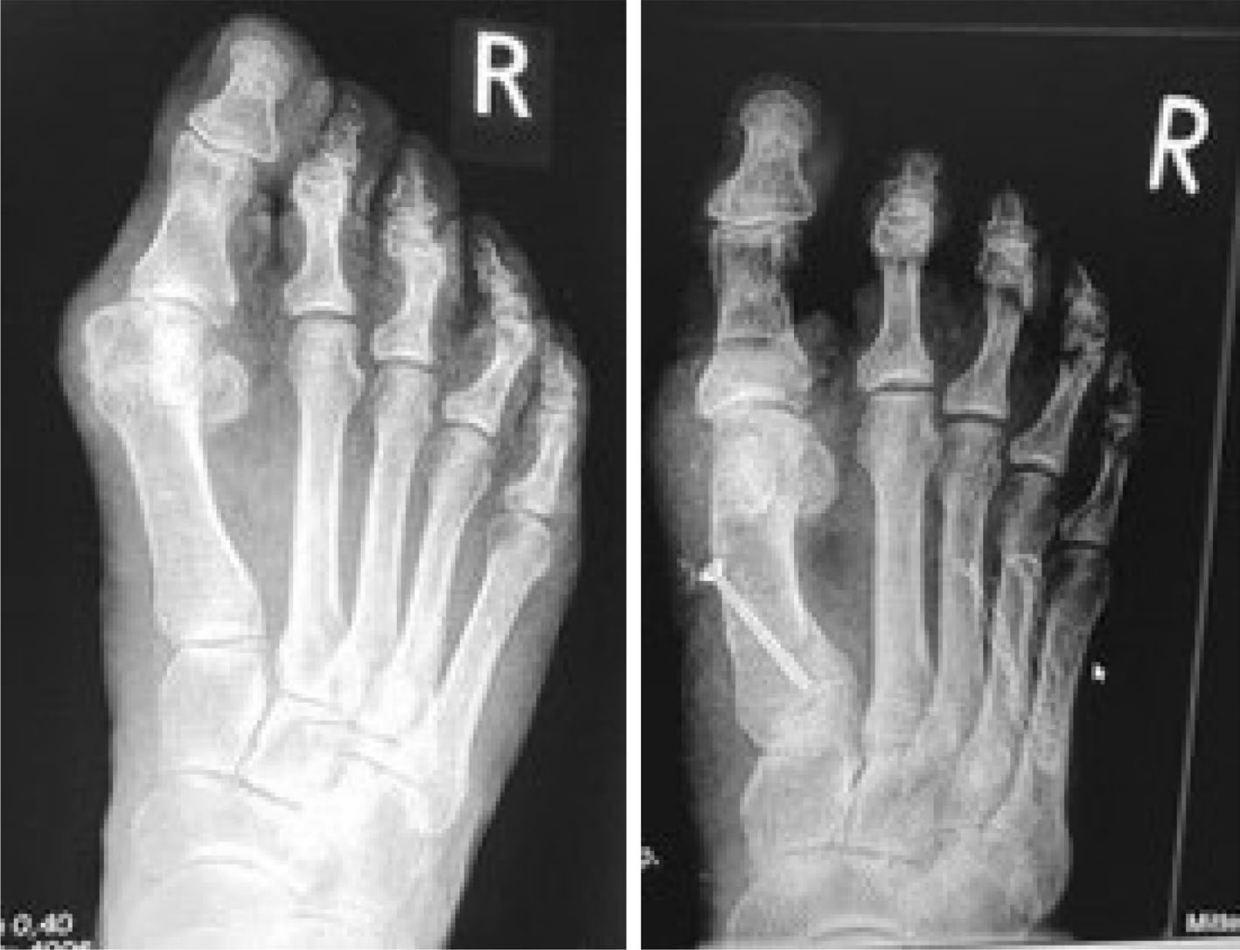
Example of pre- and post-operative X-rays following MI surgery
